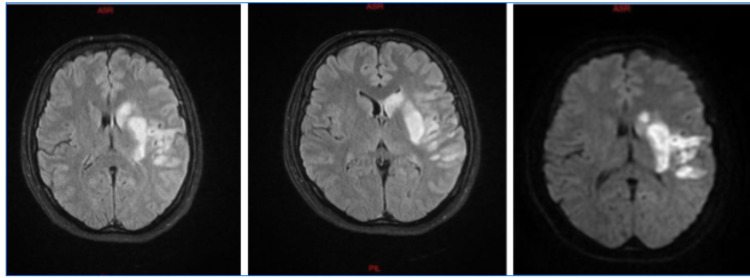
MRI results of FLAIR 1, FLAIR 2, and DWI images, respectively FLAIR - fluid-attenuated inversion recovery, DWI - diffusion-weighted image
